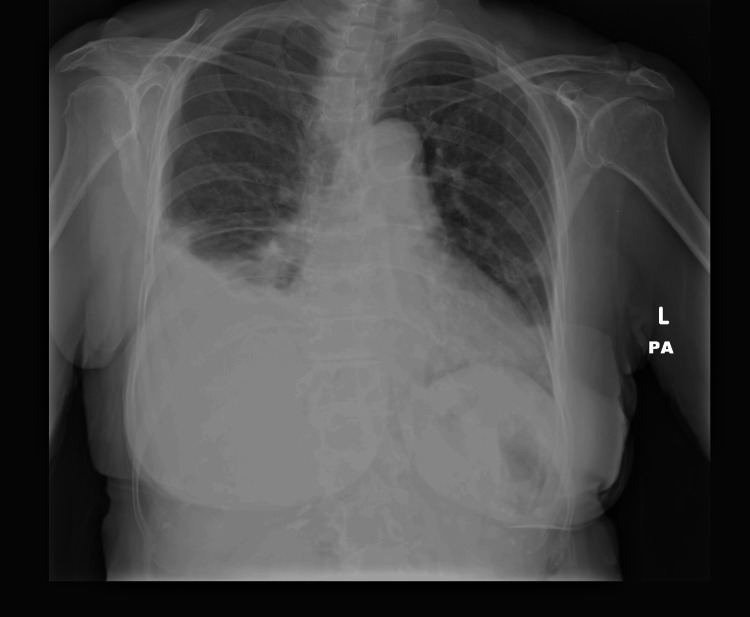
Chest X-ray. Large right pleural effusion.
